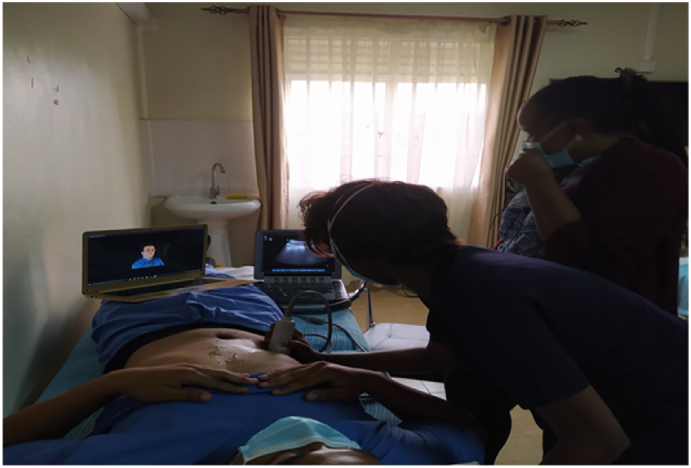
Active trainee perticipation with ultrasound probe positioning being visualised by the trainer.8.Network: Typically Mobile phone, airtime based internet is more reliable and stable as compared to office wide networks. Each station should have independent network if possible.9.Backups: As all machines can fail, backup ultrasounds and backup internet connections should be gathered. This includes keeping all electronic devices fully charged and having modalities such as UPS and power banks.10.Positioning: The laptop at each station is positioned so that the trainer has both the ultrasound image and the trainee's hand position in view. This view enables more precise directions and instruction. See Fig. 1, Fig. 6, Fig. 7.
